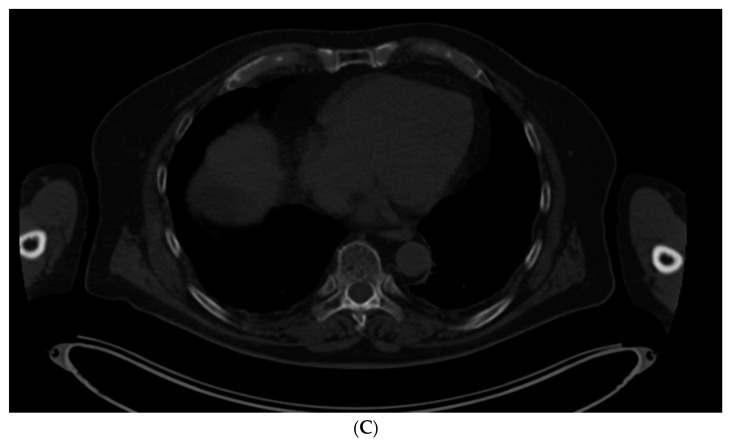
IgAk SMM patient with only one focal lesion of a thoracic vertebral body (maximum standardized uptake value (SUVmax): 15.34) depicted in FDG-PET/CT. (A: Positron emission tomography (PET) slice and B: PET/CT fused slice). Because of the absence of osteolytic lesions on the opposite CT (C), biopsy was performed in this area, revealing a focal monotype k accumulation of clonal plasma cells, upgrading the patient to symptomatic multiple myeloma (MM) requiring treatment.
